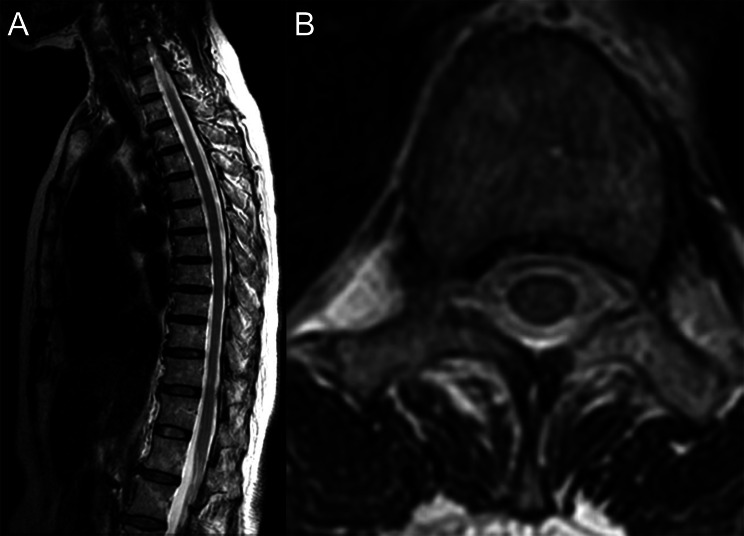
T2-weighted magnetic resonance imaging obtained 7 months after onset shows resolution of the hematoma. A) Sagittal section. B) Axial section at Th11
